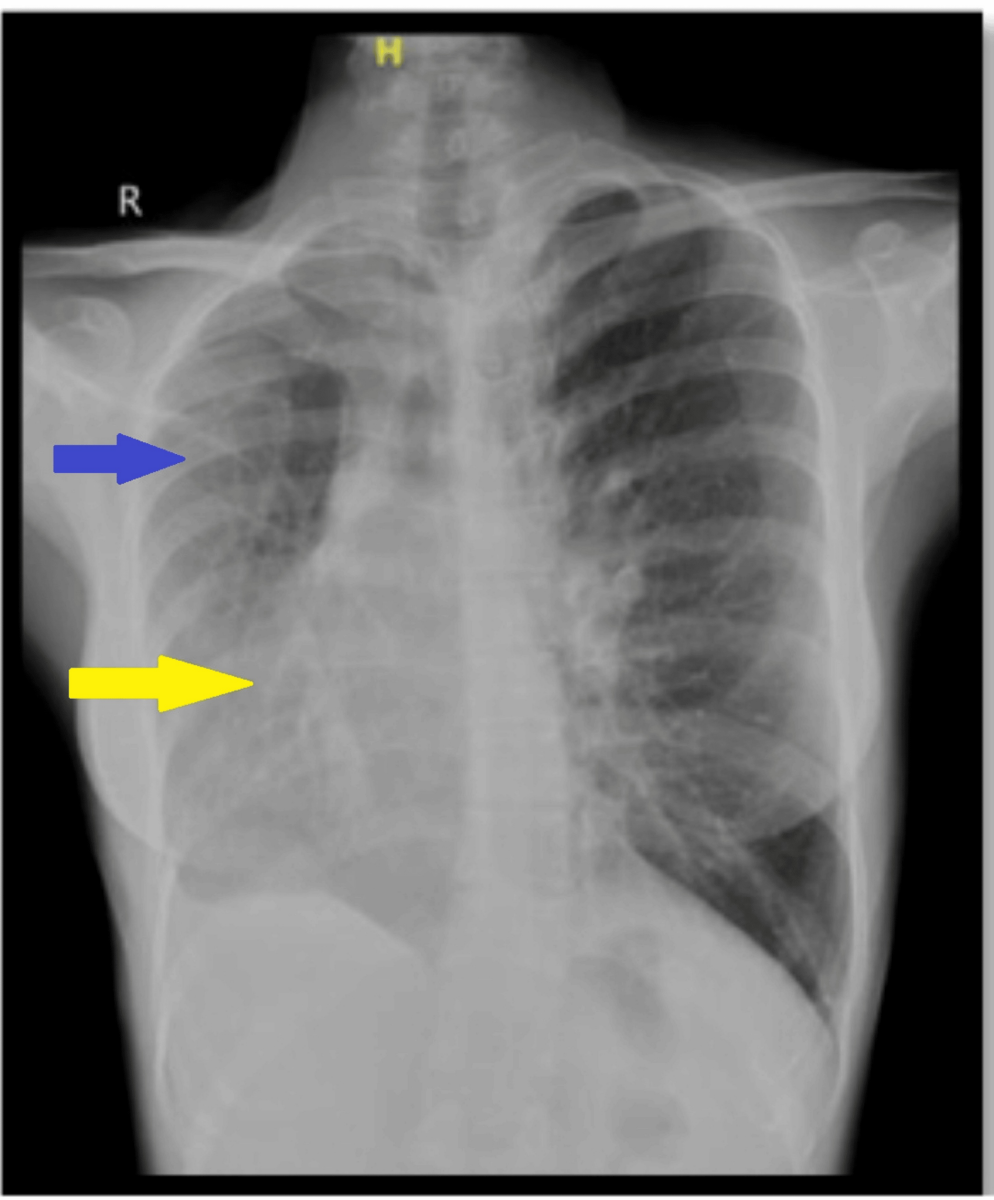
Chest X-ray- showing right-rotated heart (yellow arrow) with hypoplastic right lung field (blue arrow)
